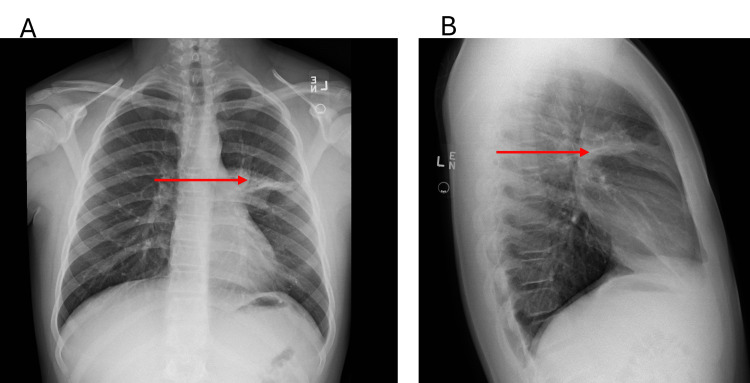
Posteroanterior (A) and lateral (B) chest X-rays on the second visit. A and B show persistent consolidation despite initial therapy on the second visit. Arrows highlight areas of consolidation.
